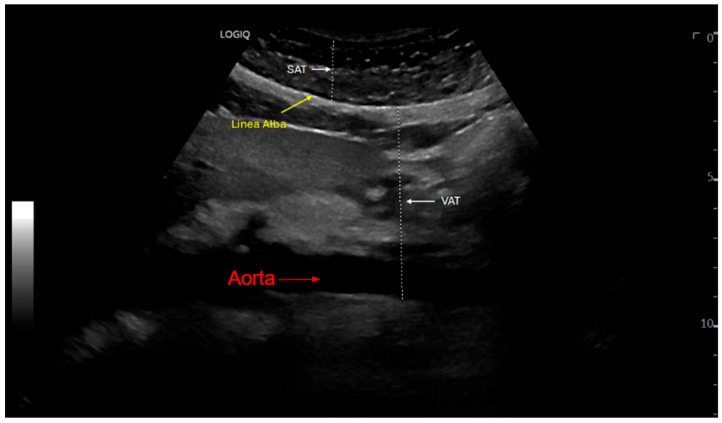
A transabdominal ultrasound image demonstrating subcutaneous and visceral adipose tissue: A transabdominal gray-scale ultrasound image of the midline upper abdomen acquired with a low-frequency (high-depth) ultrasound probe in the supraumbilical region in a longitudinal position. The longitudinal orientation of the aorta (red arrow) demonstrates that this image is acquired in the sagittal orientation. Subcutaneous adipose tissue (SAT) is measured from the deep aspect of the epidermis to the linea alba (the echogenic line marked by the yellow arrow). In this image, visceral adipose tissue (VAT) is measured from the linea alba to the posterior wall of the aorta. Figure courtesy of Jordan Perchik MD.
